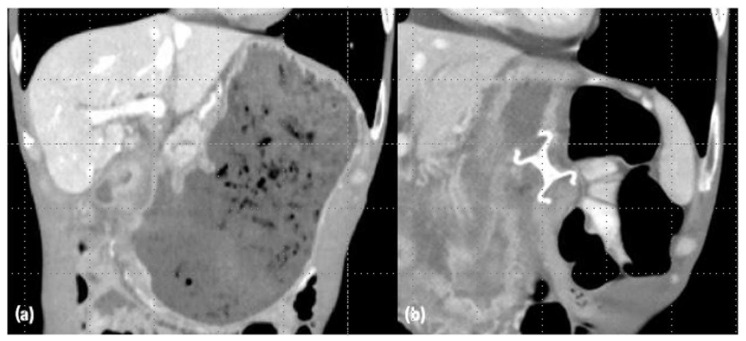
CT scan: (a) a case of significant gastrectasia due to tight neoplastic pyloric stricture; (b) same patient after positioning of EUS-GE with LAMS (visible in the image): the stomach is empty, and the stent is patent.
